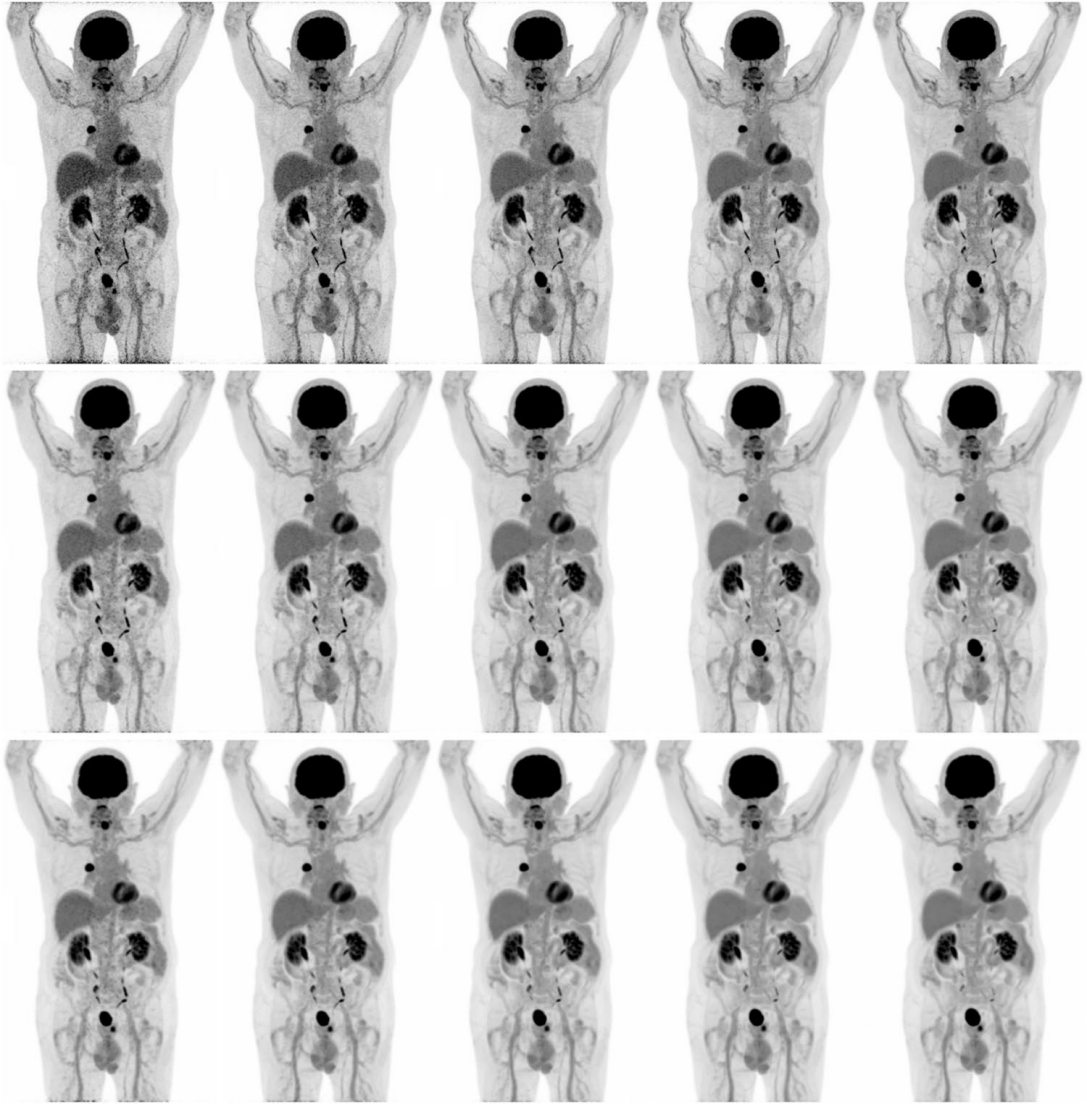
Maximum intensity projection PET images of an 80-year-old male (weight, 93 kg) with right-sided non-small cell lung carcinoma. The patient received a single injection of 279 MBq.^18^F-FDG, and PET images were acquired at 60 min post-injection. Listmode PET data were reconstructed using CLIN (top row), EARL standards 2 (middle row) and EARL standards 1 (bottom row) reconstruction settings for various scan durations: 60 s, 120 s, 240 s, 420 s and 600 s (from left to right)
